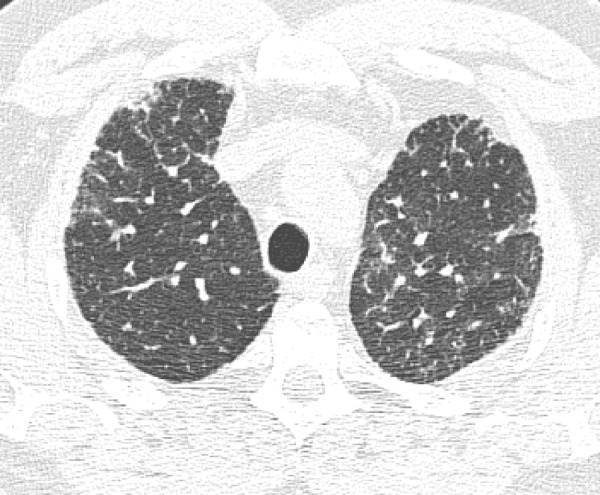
CT pattern indeterminate for usual interstitial pneumonia. (a–c) Axial inspiratory CT images demonstrate peripheral and lower lung–predominant reticular abnormality with architectural distortion, including traction bronchiectasis. However, there is slightly more ground-glass abnormality than typically seen with a confident diagnosis of a usual interstitial pneumonia pattern, and the reticular abnormality extends along the bronchovascular bundles rather than being confined to the subpleural lung.
